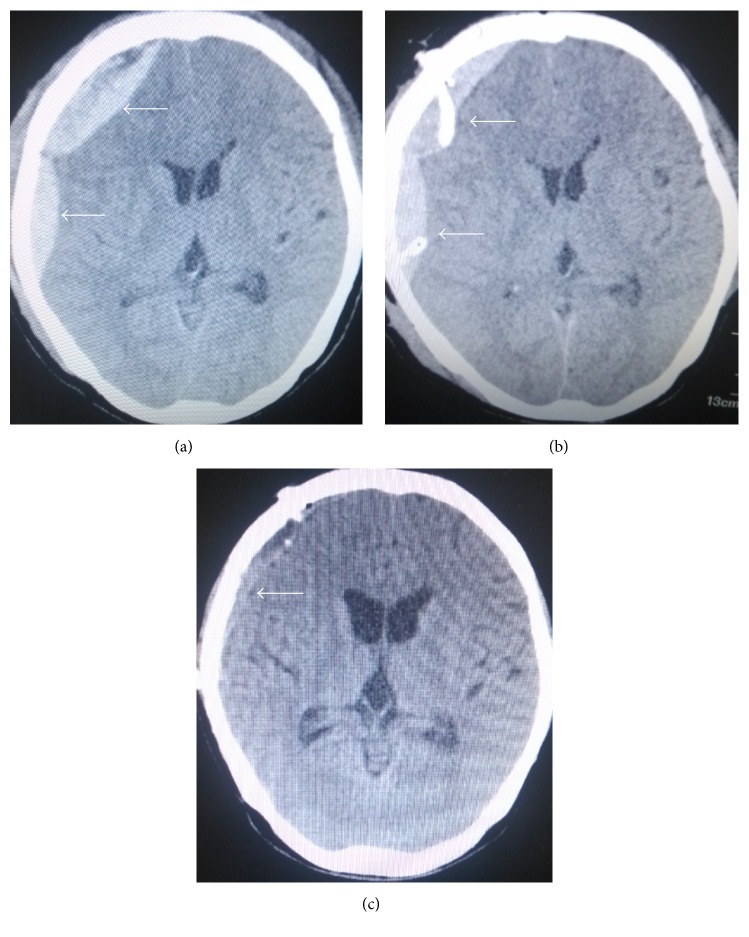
(a)–(c) Head CT images showing minimally invasive drainage treatment of acute multiple epidural hematoma at the right temporal-parietal region prior to and after surgery. (a) Head CT scan image prior to surgery. (b) Head CT scan reexamined immediately after surgery; hematoma volume at the parietal region was markedly reduced. Arrow indicates hematoma and drainage tube. (c) Five days after surgery, hematoma was almost cleared and the drainage tube was pulled off.
